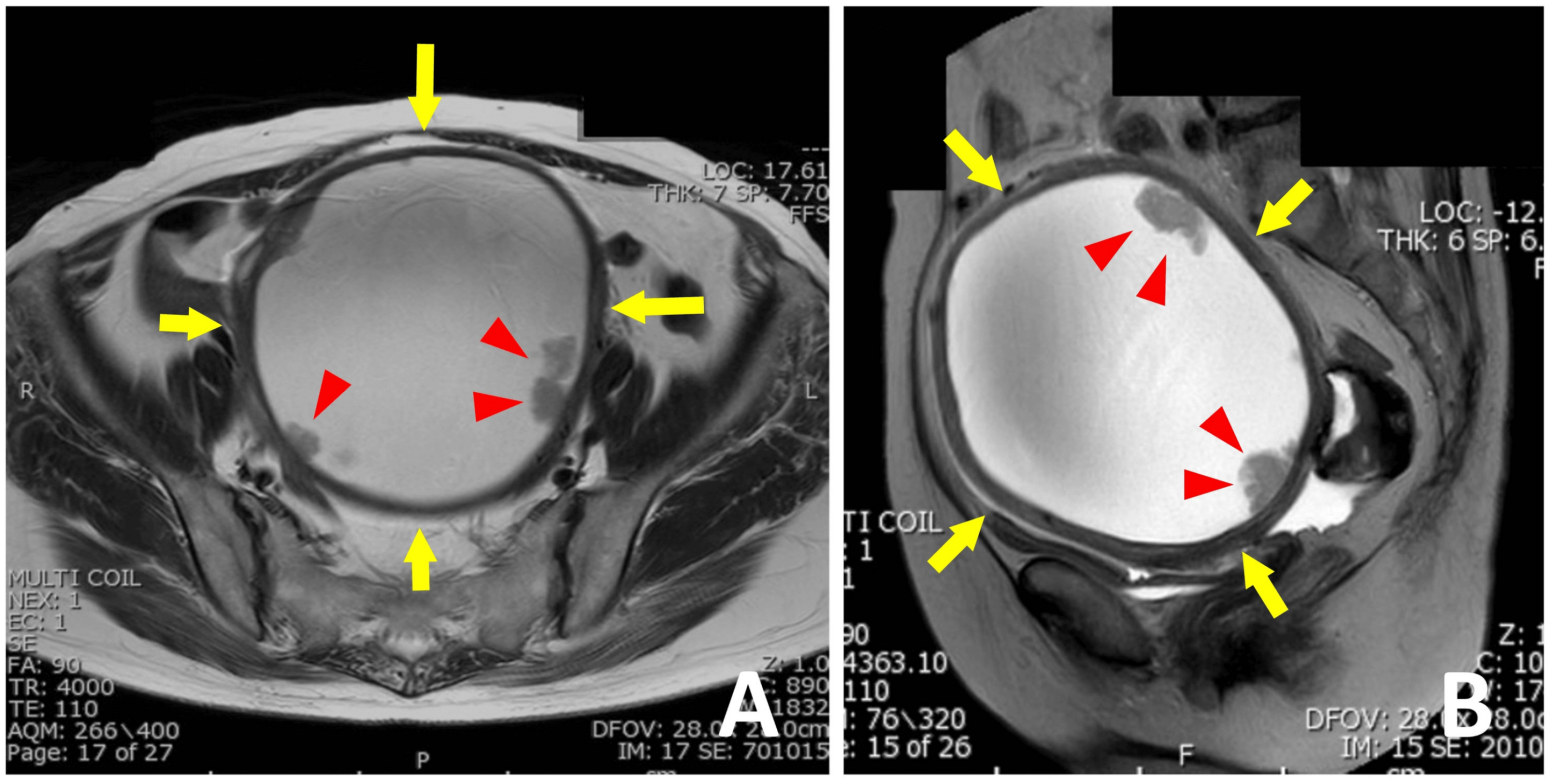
Magnetic resonance imaging of the pelvic cavity. Axial (A) and sagittal (B) T2-weighted MR images show a cystic tumor (arrows) with solid papillary components (arrowheads) protruding into the cyst lumen.
